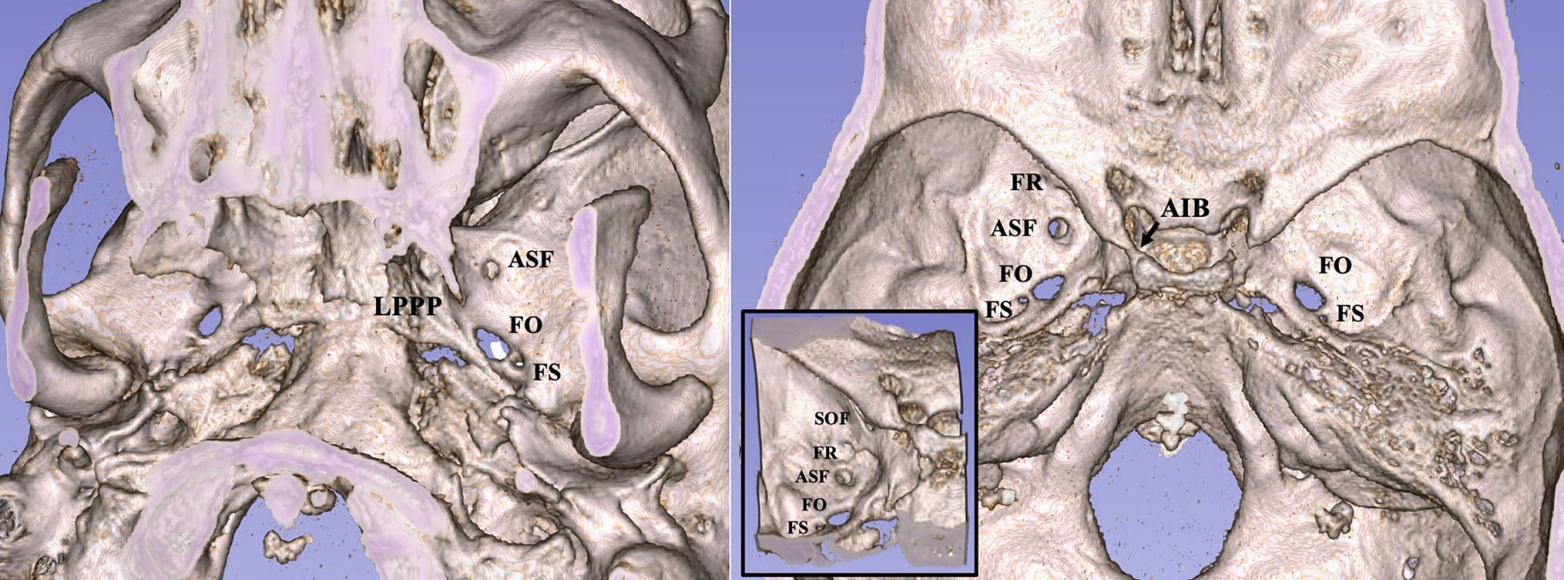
The computed tomography depicts the accessory sphenoidal foramen (ASF) of the middle cranial fossa. Three-dimensional reconstruction of the CT, left figure: extracranial view of the middle cranial fossa depicting the ASF opening into the infratemporal fossa. Right figure: intracranial view. Small figure: intracranial view with the superior orbital fissure (SOF). *FO* foramen ovale, *FS* foramen spinosum, *SEF* sphenoidal emissary foramen, *FR* foramen rotundum, *AIB* anterior interclinoid bar
